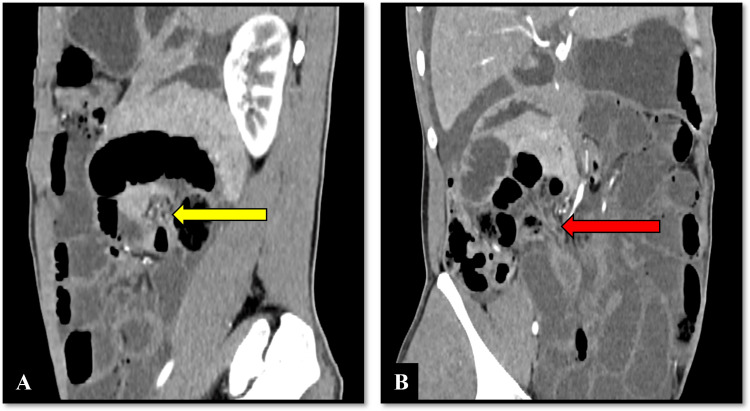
Whirlpool sign and small bowel volvulus within the right paraduodenal hernial sac Parasagittal (A) and curved planar reconstruction (B) sections of the CECT abdomen show a swirl of mesenteric vessels (yellow arrow) noted near the fossa of Waldeyer, indicating volvulus. B: Small bowel loops are noted entering and exiting the hernial sac (red arrow) causing closed-loop obstruction with volvulus. CECT: contrast-enhanced computed tomography
